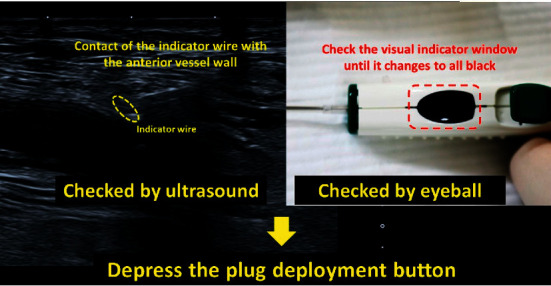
Ultrasound finding when we should depress the plug deployment button.
